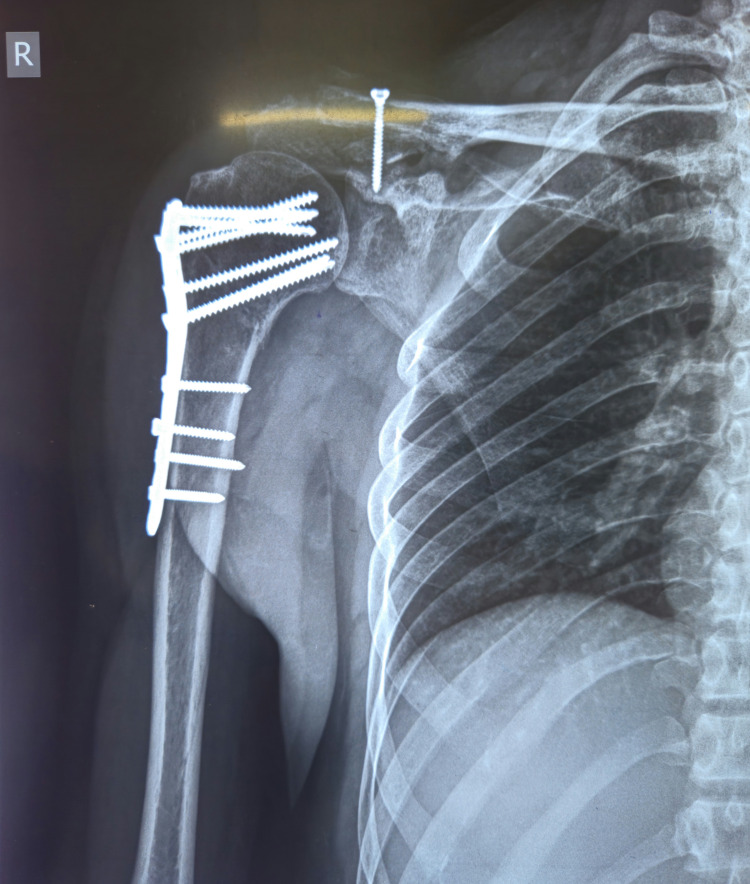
Postoperative radiograph of the affected shoulder at the 12-month follow-up showing complete union of the proximal humerus fracture with intact implant, maintained reduction of the glenohumeral joint and acromioclavicular joint stabilized with a coracoclavicular screw, and a healed scapular spine fracture.
